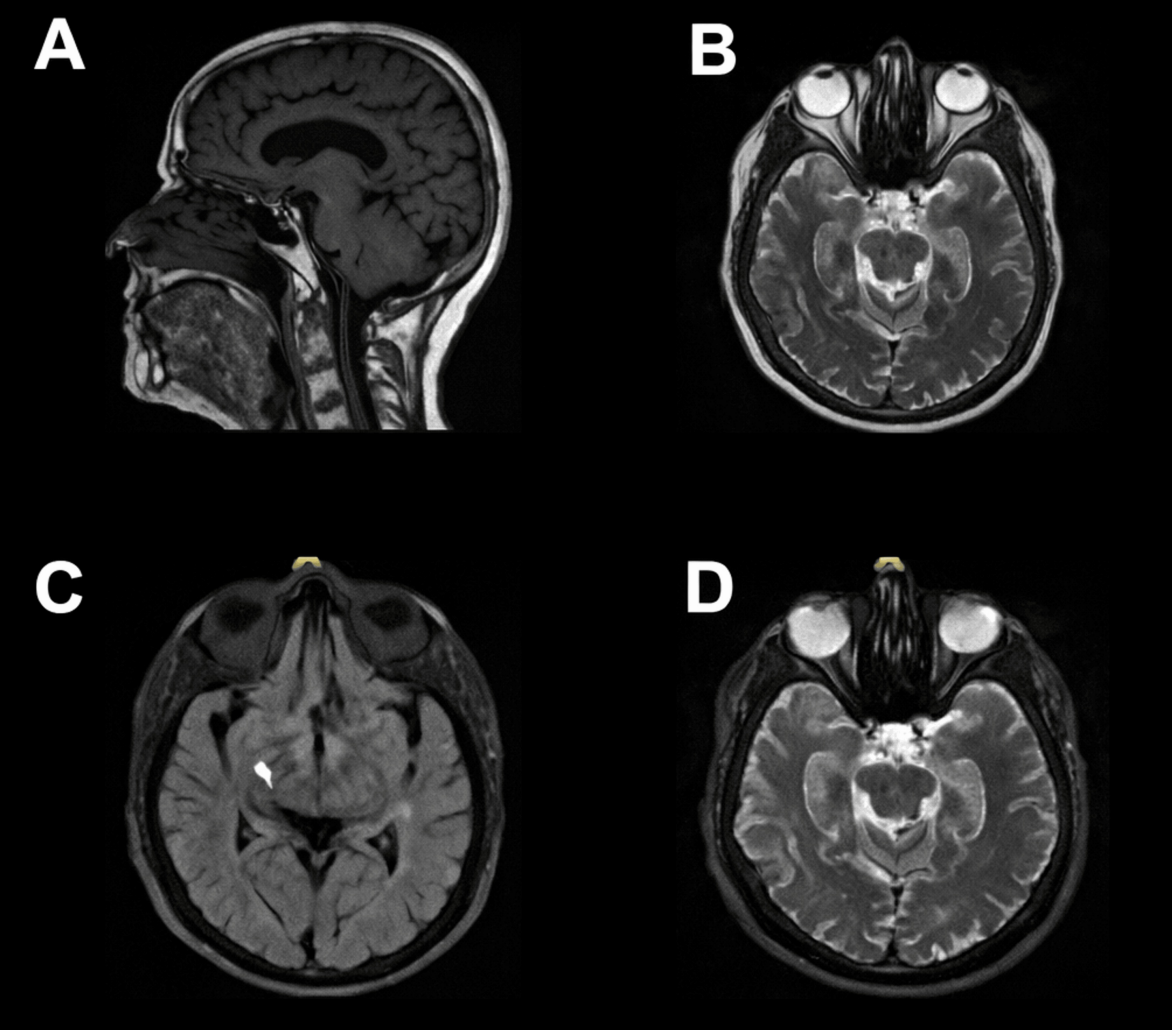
Three-month follow-up MRI demonstrating marked interval reduction of the prior hemorrhagic lesion. A. Sagittal T1-weighted MRI showing normal midline structures with subtle residual signal abnormality at the site of the prior lesion. B. Axial T2-weighted MRI demonstrating symmetric appearance of the midbrain with mild residual hyperintensity in the region of the previous hemorrhage. C. Axial FLAIR sequence showing a small residual focus of signal alteration in the left perimesencephalic region. D. Axial T2-weighted MRI with minimal linear hyperintensity in the area corresponding to prior hemorrhage. FLAIR, fluid-attenuated inversion recovery
